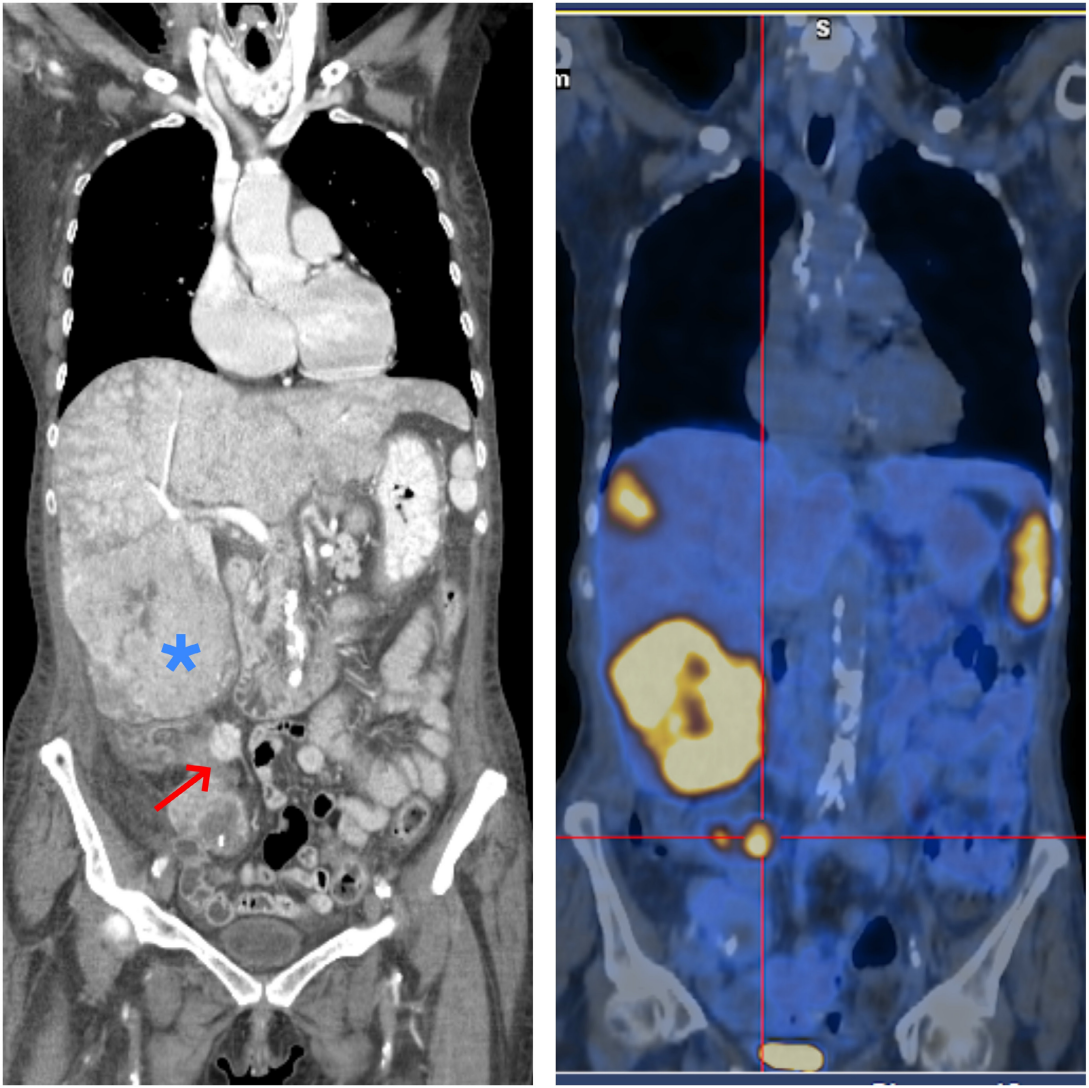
LEFT: CT scan showing a mass on the terminal ileum (red arrow) and diffuse liver metastasis (blue star). RIGHT: 68‐Ga DOTATOC PET/CT scan revealing the ileal and liver lesions as expressing somatostatin receptors.
